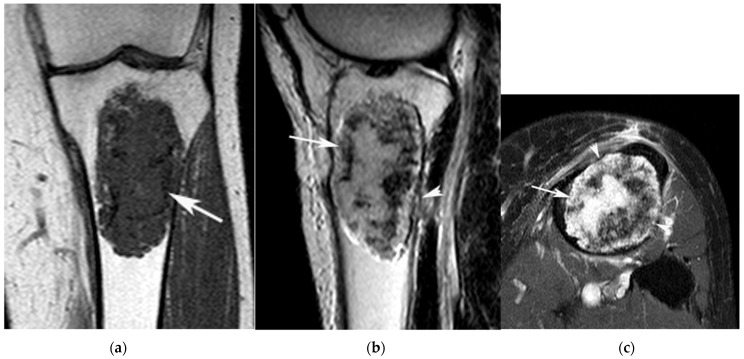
A 56-year-old female with an ACT in the left proximal tibia. (a) Coronal T1W TSE, (b) sagittal T2W FSE and (c) axial SPAIR MR images show a chondral tumour (arrows) centred on the proximal metaphysis of the tibia. The lesion fills the medullary cavity and results in multifocal deep endosteal scalloping (arrowheads (b,c)) but does not cause in any bone expansion or cortical destruction.
